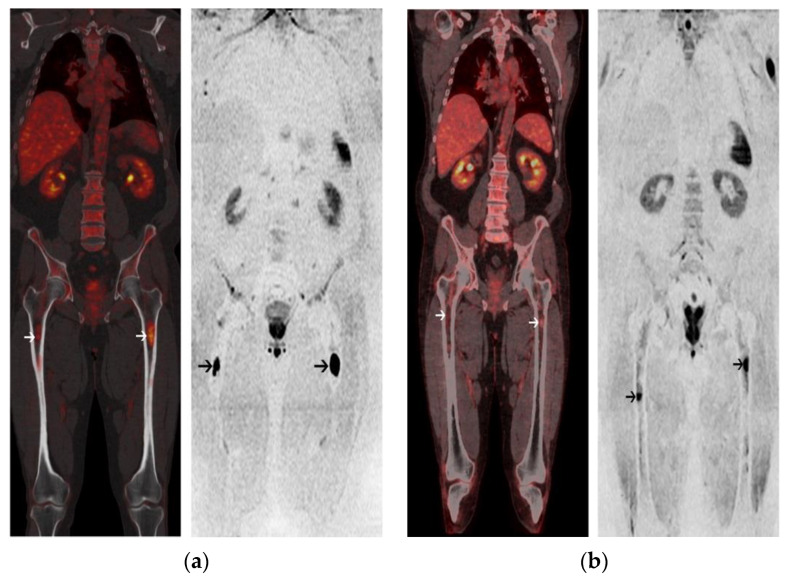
A 63-year-old male with Kappa Light Chain MM. (a) Coronal image of 18-FDG PET/CT at baseline (left panel) shows 2 focal lesions (FL) within the femurs (white arrows). Corresponding coronal image of baseline WB-DW-MRI (right panel) shows 2 FL with restricted diffusion within the femurs; (b) Post-ASCT 18-FDG PET/CT is negative and coronal image (left panel) shows a regression of uptake of the femoral FLs (FL SUVmax < liver uptake) (white arrows). Post-ASCT WB-DW-MRI shows a regression of the extent of the femoral FL (black arrows) associated with a decrease of the diffusion restriction and a significant increase of the ADC value from baseline (>40%). Patient was classified as low-risk by both imaging modalities and is in complete remission after 33 months of follow-up.
